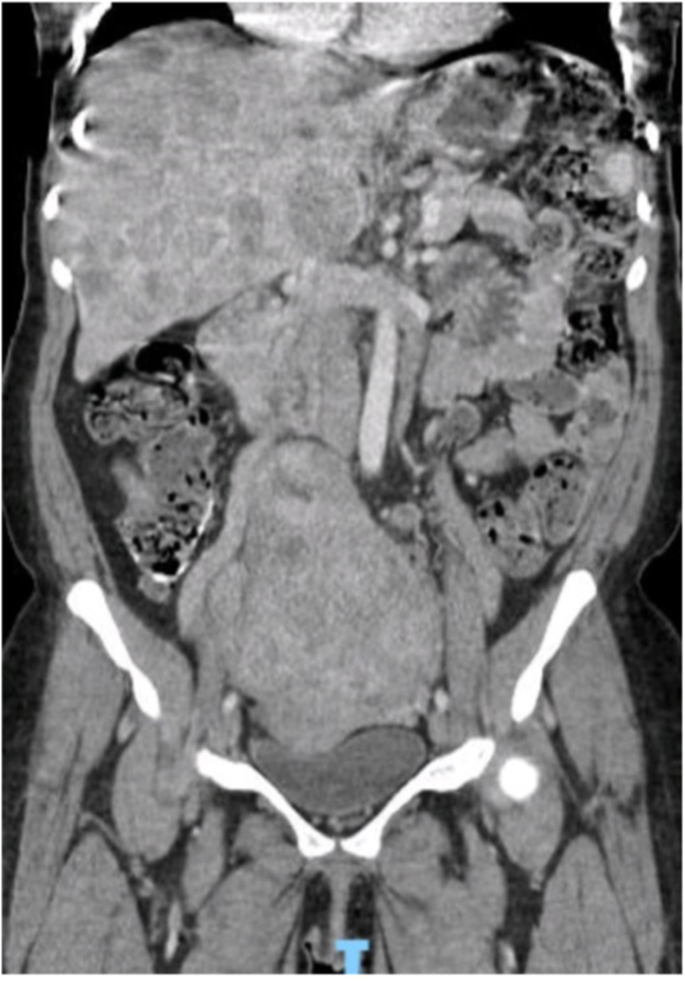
Coronal plane of CT scan of abdomen.
